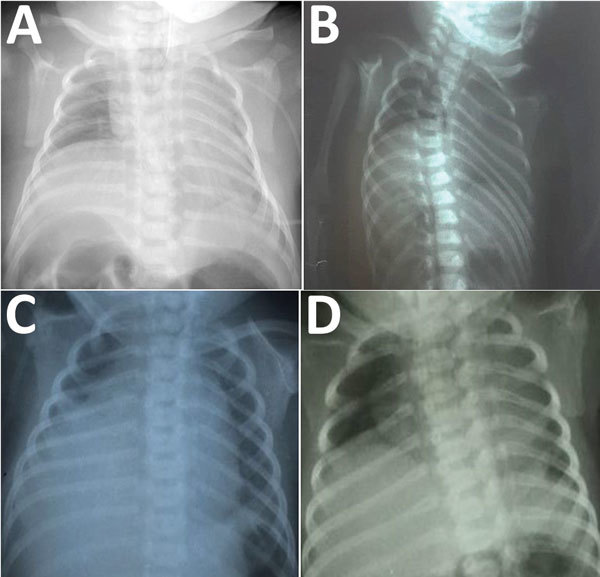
Chest radiographs of infants with congenital Zika syndrome, demonstrating elevation of the right hemidiaphragm. Panels A–D represent patients 1–4, respectively. In each instance, only the right hemidiaphragm was noticeably elevated. All patients also had arthrogryposis (including talipes equinovarus) and died from complications related to progressive respiratory failure.
